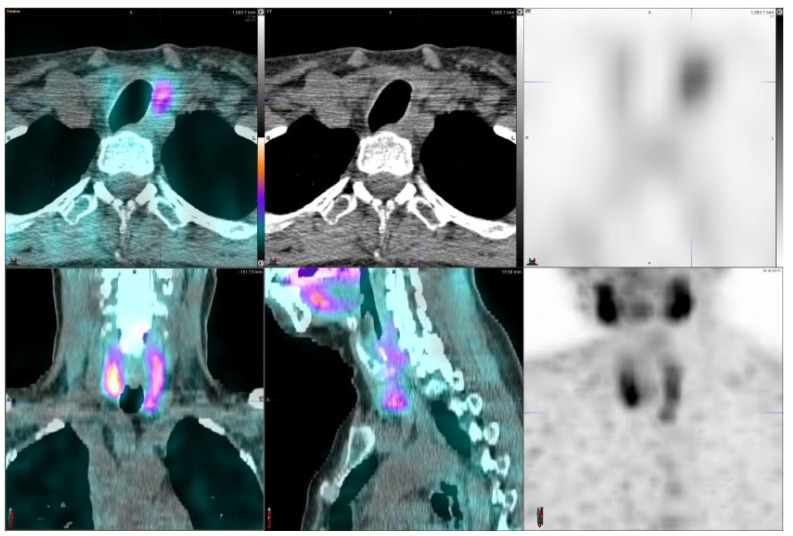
A 65-year old female with hyperparathyroidism. A left inferior parathyroid adenoma, weighing 123 mg and an intrathyroid nodule on the right are seen. Her S-PTH concentration was 154 ng/L and S-Ca 10.9 µg/L. The SUVmax values of the left-sided adenoma were 5.1 (CGZAS) and 3.1 (CGAS), respectively. Visually, this uptake was weak (1 < TBR < 2) and lesion diameter on CT was 7 mm. The intrathyroid nodule demonstrated higher uptake. This is a potential pitfall in adenoma imaging, but can be avoided by multimodality practice.
